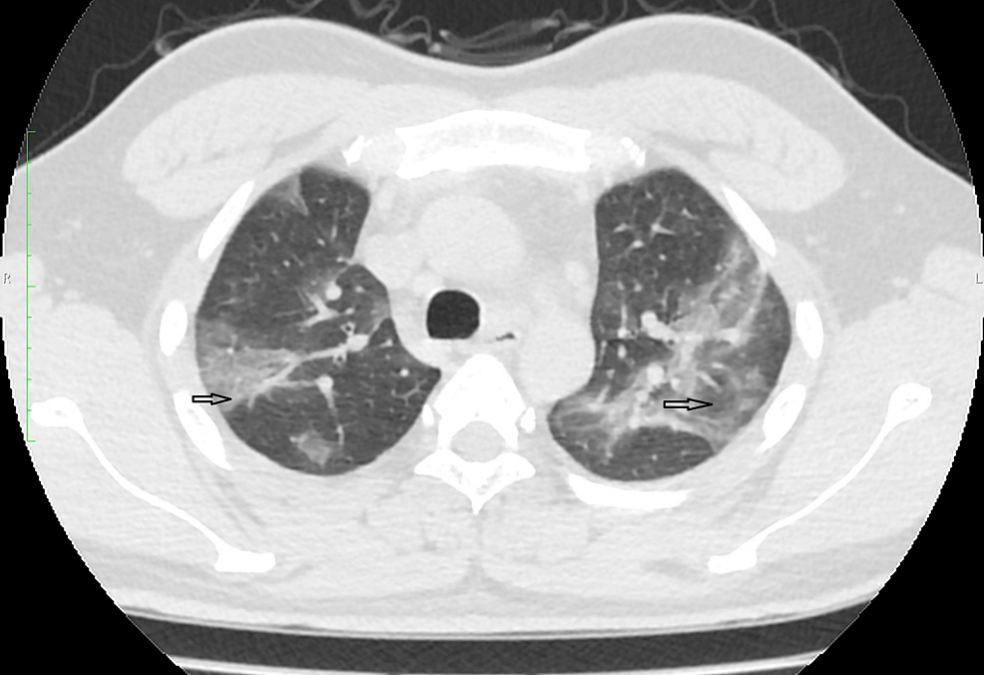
CT Chest Showing Diffuse Crazy Paving Pattern, Consolidation, and Septal Thickening
